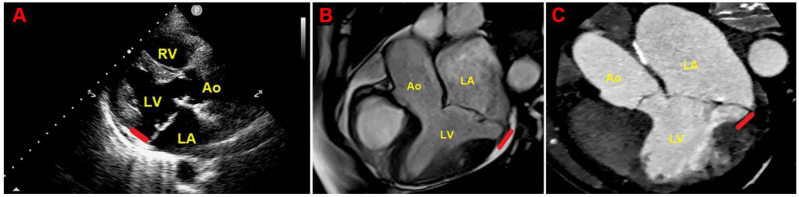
Example of MAD assessment at end-systole from echocardiographic parasternal long-axis view (A), from CMR-derived three-chamber view (B) and from multiplanar reconstructed three-chamber view on CCTA (C), in individual with bileaflet MVP. Ao, aorta; CCTA, cardiac computed tomography angiography; CMR, cardiac magnetic resonance; LA, left atrium; LV, left ventricle; MAD, mitral annular disjunction; MVP, mitral valve prolapse; RV, right ventricle. The red lines indicate the MAD distance.
